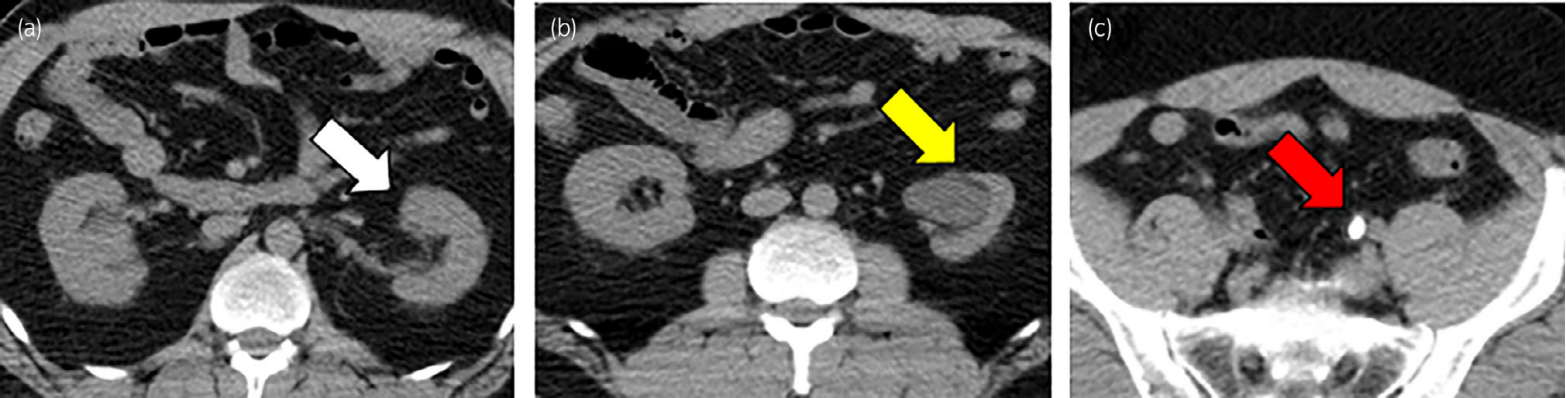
A follow‐up CT of a parotid carcinoma taken 10 months before the renal rupture showed (a) the upper half of the kidney retaining its renal cortex morphology (white arrow), while (b) the lower half of the kidney was atrophied (yellow arrow). (c) Ureteric calculus were identified upstream from the confluence of the lower half of the renal ureter (red arrow).
